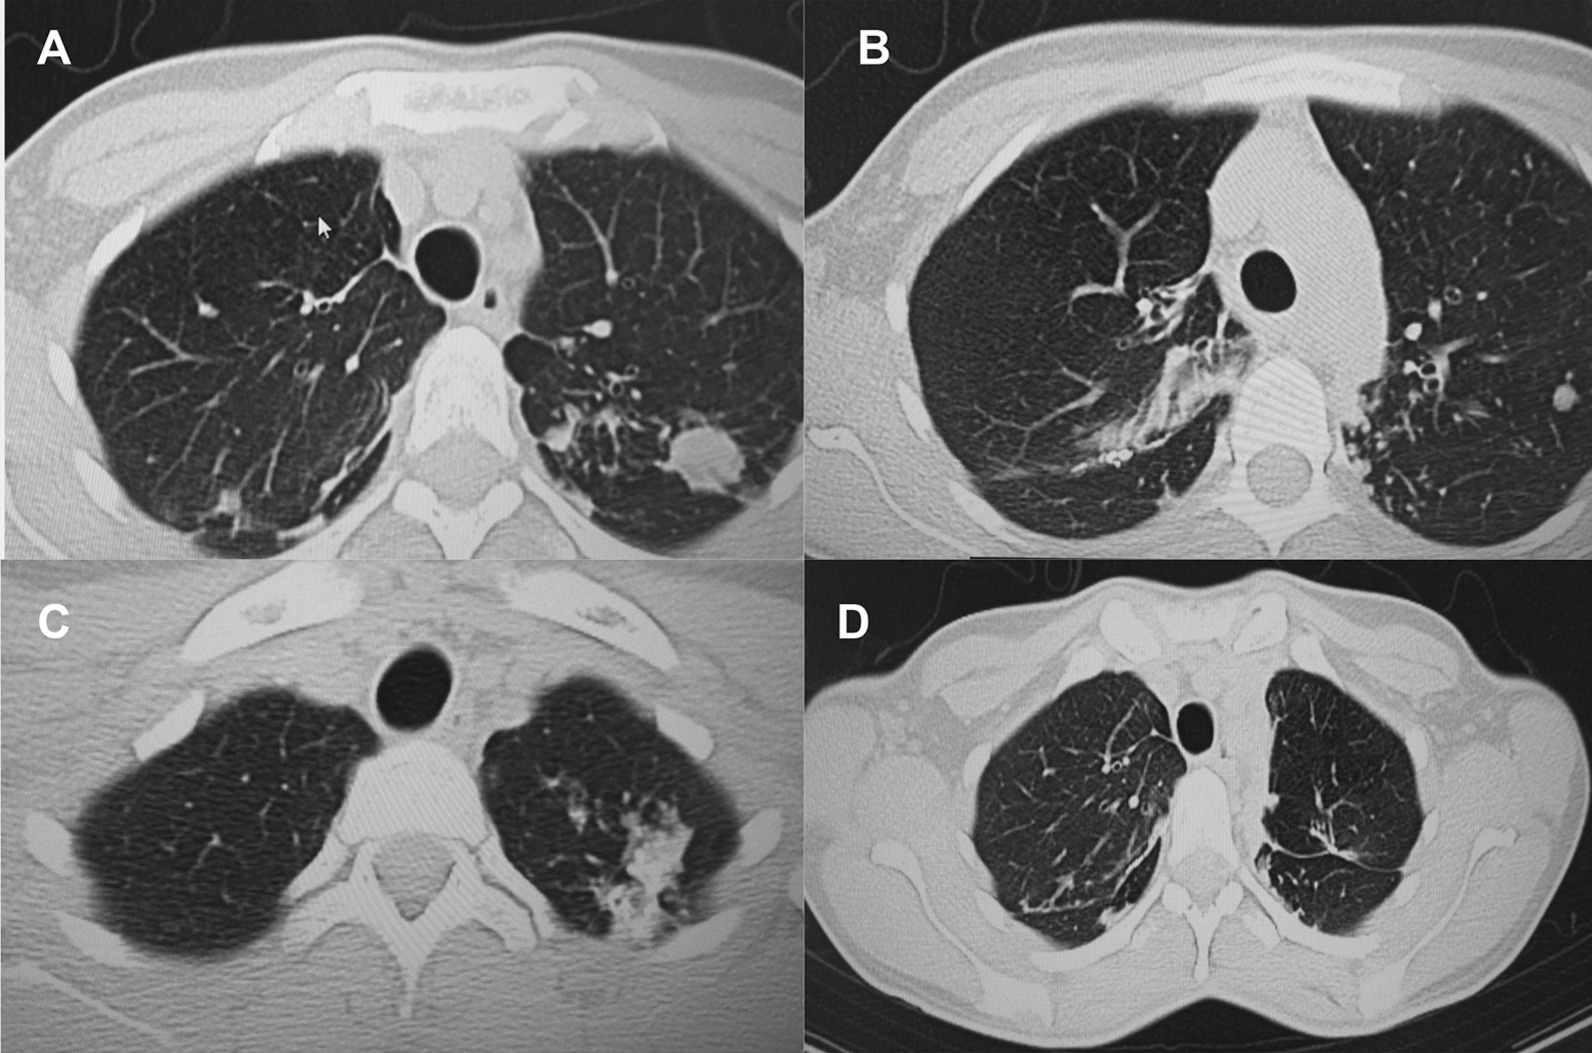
A–C Follow-up CT scan after first adjunctive surgery showing postoperative changes of the right lung and radiological changes in the left lung, that were unchanged compared to the initial CT. D Final CT scan showing normal postoperative changes with no cavities as previously seen
